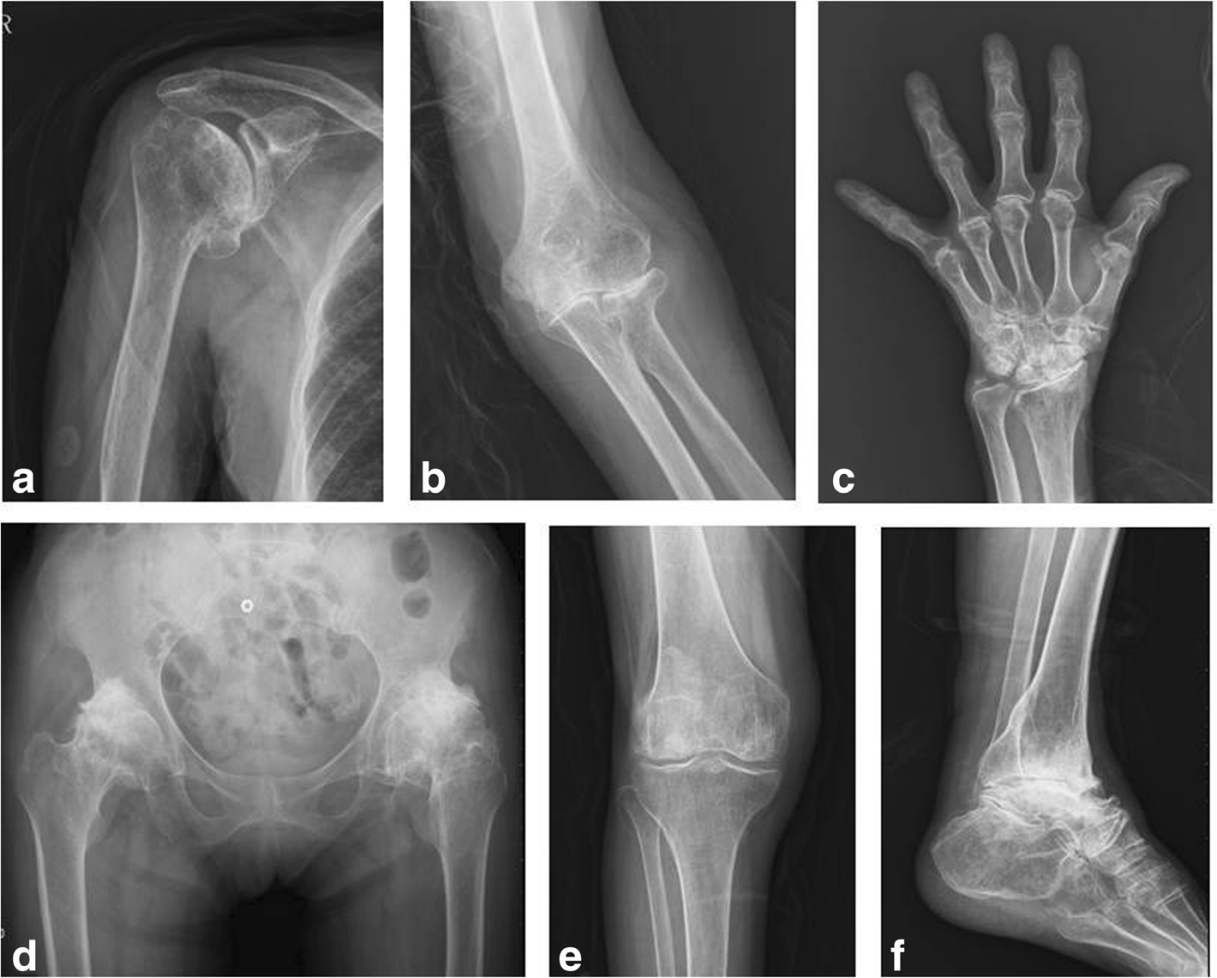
X-rays display the multiple joints affected in an elderly patient with end-stage KBD: a shoulder, b elbow, c wrist and fingers, d hip, e knee and f ankle
